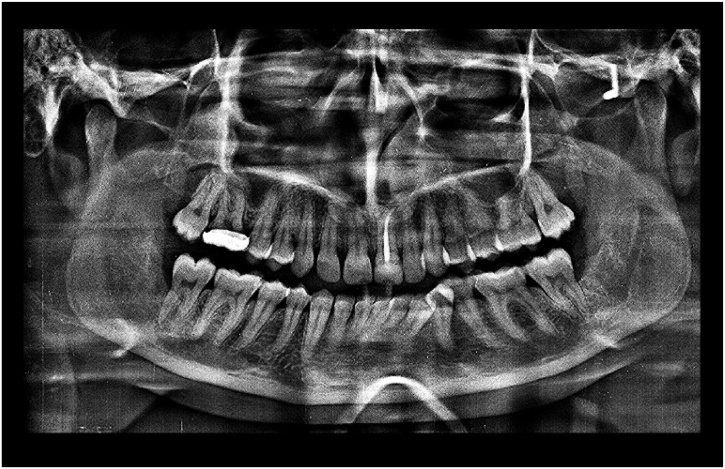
Six Months Postoperative Orthopantomograph.
